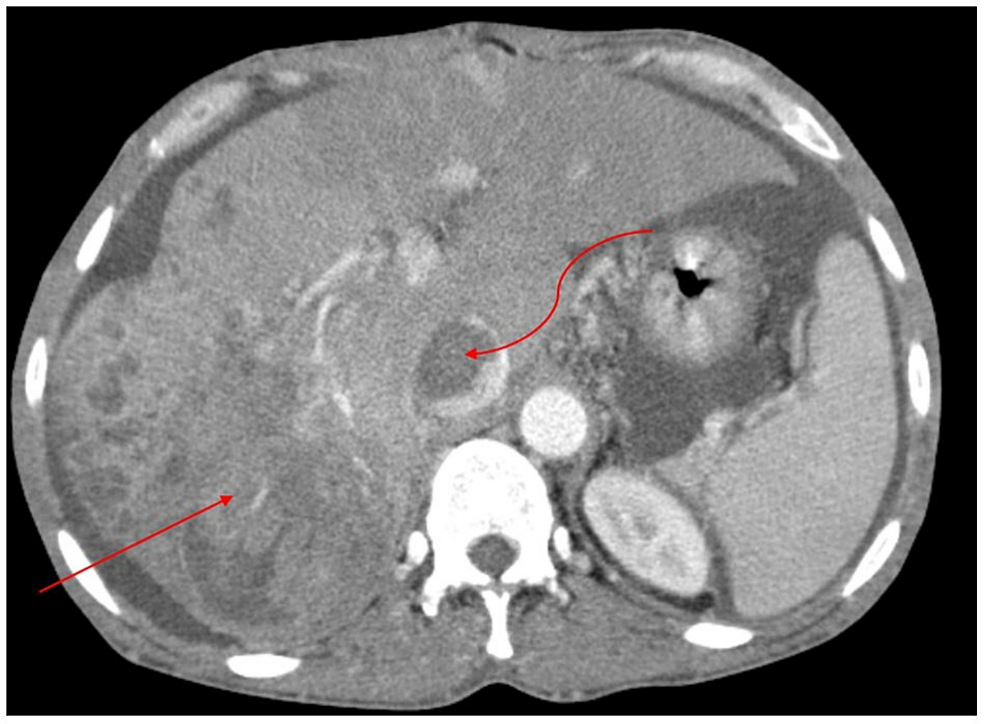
Axial early portal venous phase post-contrast CT image through the liver shows a heterogeneous mass in the right hepatic lobe, with areas of contrast washout (thin, straight arrow) consistent with hepatocellular carcinoma. The mass is seen invading the hepatic venous drainage into the inferior vena cava (curved arrow).
